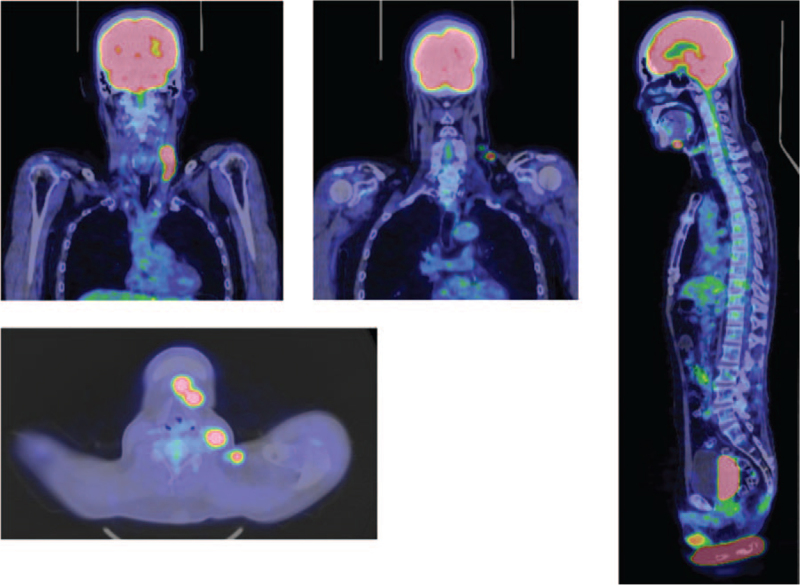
PET-CT In the regions of the bilateral accessory nerves, left lower inner deep neck, left submandibular, and bilateral supraclavicular fossae, lymphadenopathy, and abnormal FDG accumulation were observed. The left supraclavicular lymphoma lesion had shrunk compared to the lesion 8 months earlier, but multiple lymphoma lesions newly appeared. In addition, there is an increase in accumulation in the cervical spinal cord, and there is a region where the increase is particularly strong at the C4 level. These findings suggest the presence of cervical cord infiltration. There is also localized abnormal accumulation in the lower thoracic spinal cord, which might have infiltrated. FDG = fluorodeoxyglucose, HTLV-1 = human T-cell leukemia virus type 1, PET–CT = positron emission tomography–computed tomography.
